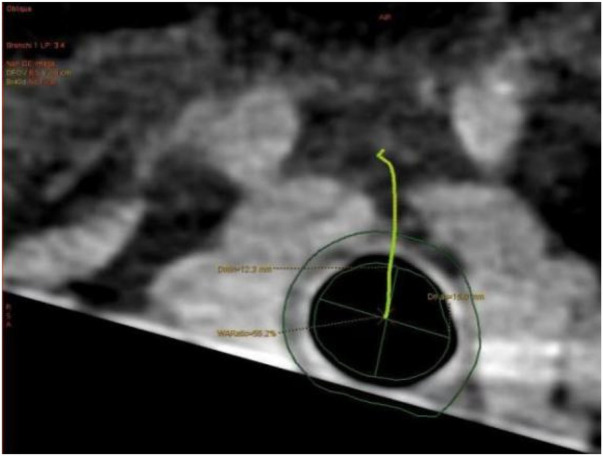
Shortest diameter of the cricoid cartilage observed on chest CT.
